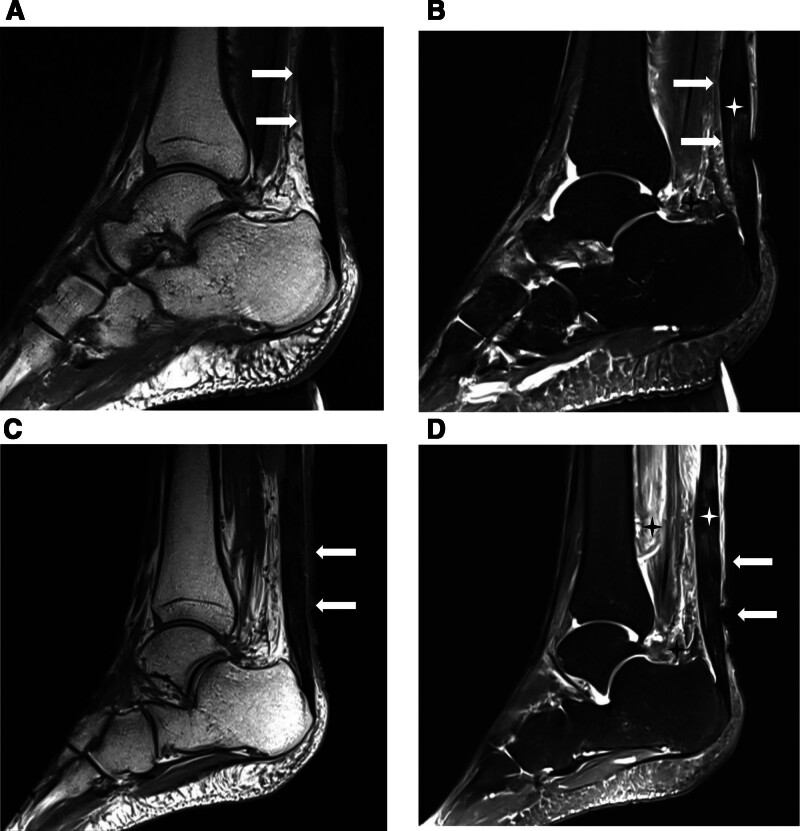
MRI findings of bilateral ankle joints. The bilateral Achilles tendons demonstrate symmetrical fusiform swelling (arrows) with signal abnormalities (white asterisks). Diffuse abnormal signal shadows (black asterisks) are observed in the soft tissues of the distal lower legs and those surrounding the ankle joints, consistent with acute Achilles tendinopathy accompanied by peritendinous inflammatory changes (A: left T1-weighted imaging; B: left T2-weighted fat-suppressed imaging; C: right T1-weighted imaging; D: right T2-weighted fat-suppressed imaging). MRI = magnetic resonance imaging.
